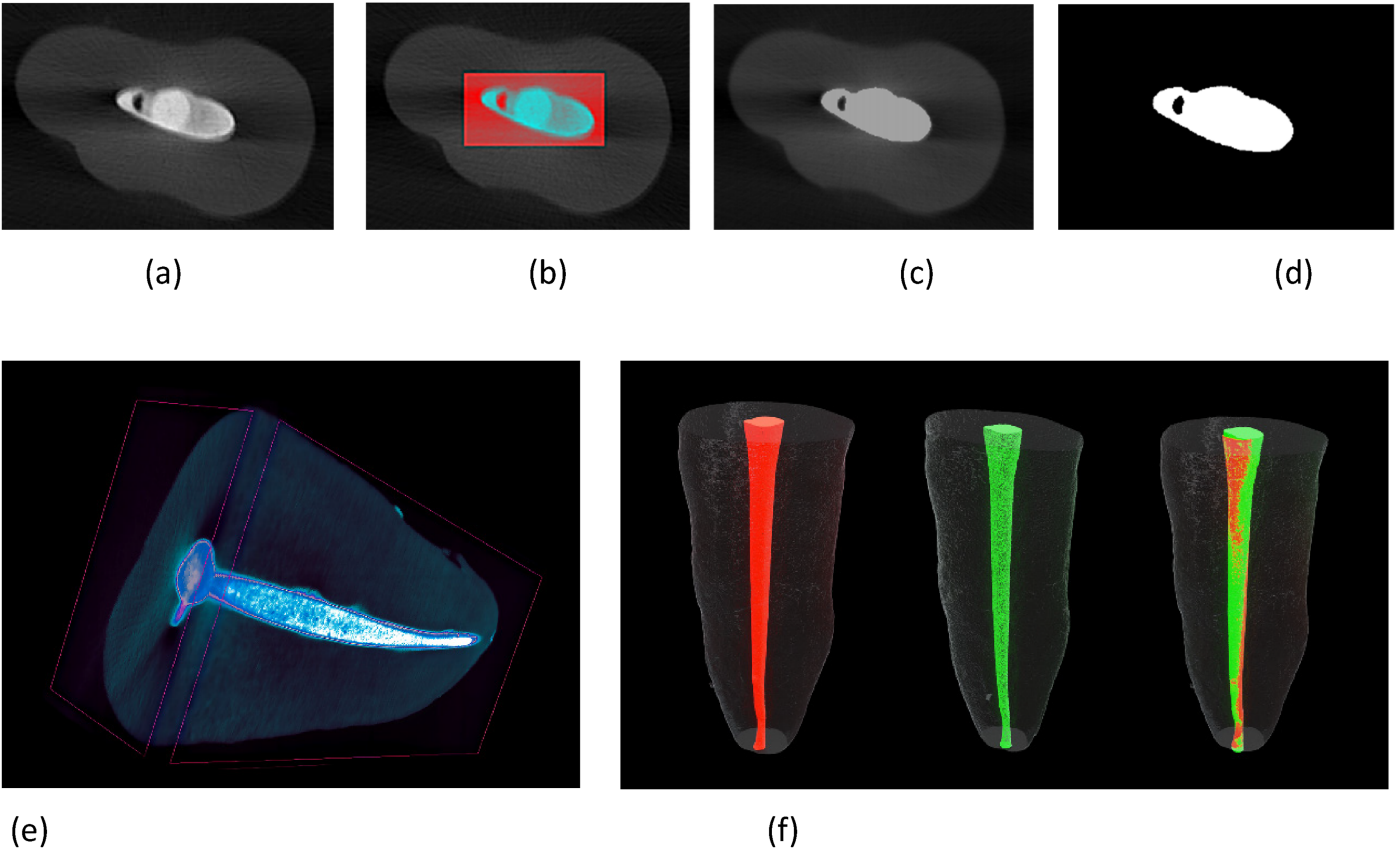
mirco-CT scan reconstruction images show voids (a) cross-sectional images, (b) selection of region of interest, (c) automatic segmentation, (d) binarization removal of dentine, (e) reconstruction of 3D model after root filling, (f) registration of 3D model before and after root filling: red, after root canal preparation; green, after root filling.
